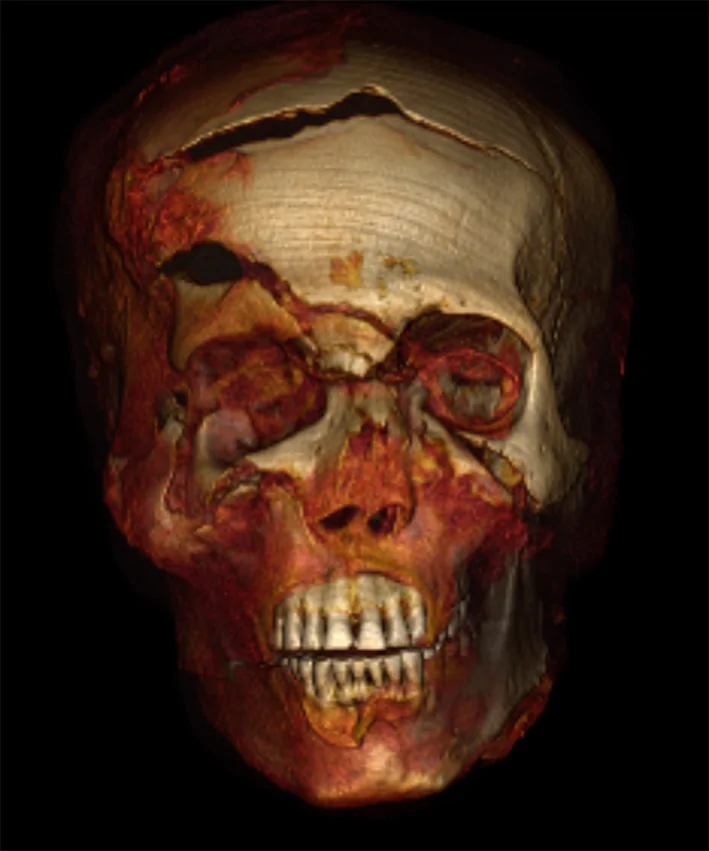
Three-Dimensional CT image of head of Seqenenre in frontal projection showing multiple craniofacial injuries: a gaping fracture of the frontal bone; fracture above the right eyebrowe; blunt trauma caused comminuted fractures of the nose, right orbit, and right zygoma; and a small perforating wound overlying the right cheek caused by fragments of the fractured zygoma.
